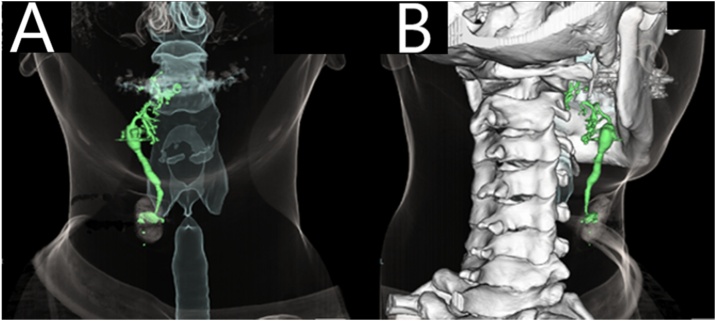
Cervical three-dimensional computed tomography fistulography. (A) The fistulous tract extends from the right lateral neck surface to the floor of the mouth. (B) A dendritic spread of contrast is observed at the root of the tongue.
